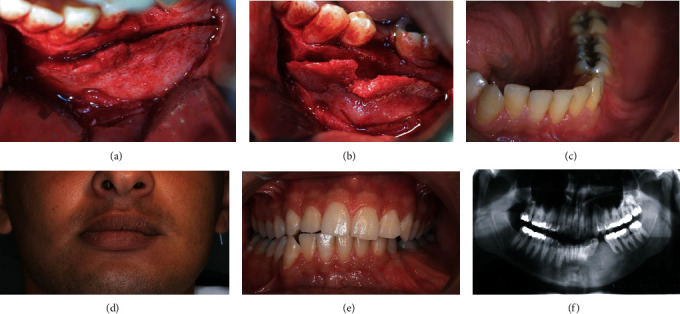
(a) Intraoperative view for the surgical approach. (b) The excised bone with respect to the mental foramen. (c) Healing of the soft tissue one month later. (d) Facial stability after 5 years following the second surgery and 15 years after the first surgery. (e) Clinical appearance of the soft healing after 15 years of the first surgery. (f) Panoramic X-ray after 15 years of the first surgery.
